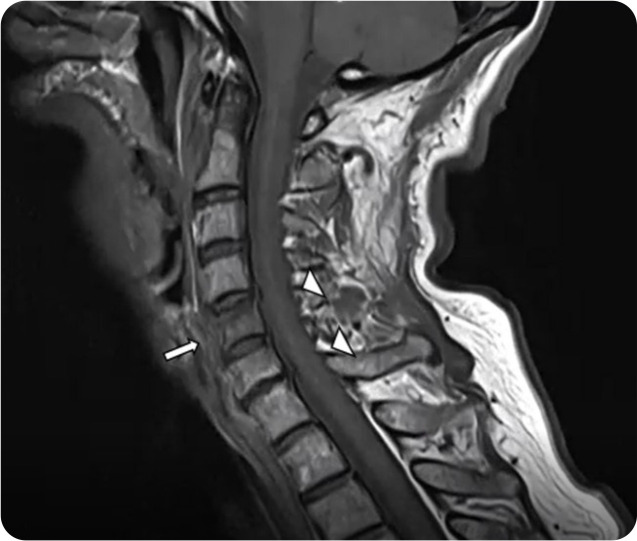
Magnetic resonance imaging T1w sequence: hypointense signal in C5 (white arrow) and degenerative structural changes in C4-C5, C6-C7 (white arrowheads).
